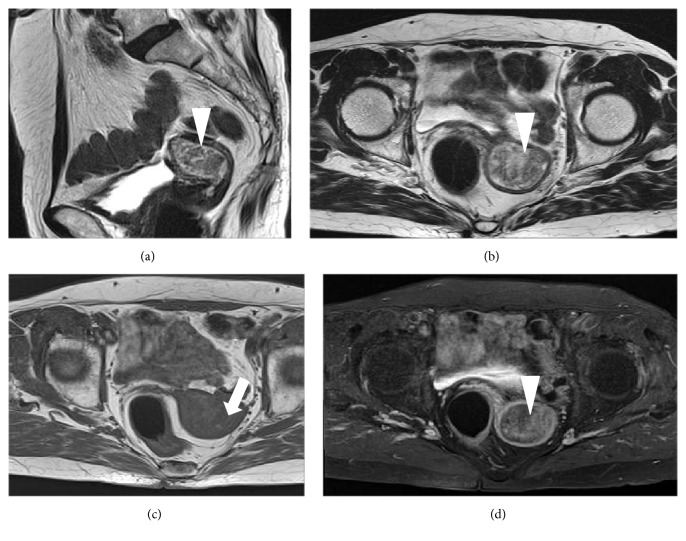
Magnetic resonance imaging of this patient. (a) Sagittal T2-weighted images (T2WI), showing an intrauterine tumor (triangle), which exhibited punctate heterogeneous hyperintensity or islands of isointense-to-hypointense signals. (b) View of the tumor (triangle) on axial T2WI. (c) Axial T1-weighted images (T1WI), showing that the tumor was detected as a hypointense signal with focal areas of high signal intensity (arrow), suspected of being hemorrhagic foci. (d) Axial contrast-enhanced T1WI showing a little tumor enhancement (triangle).
